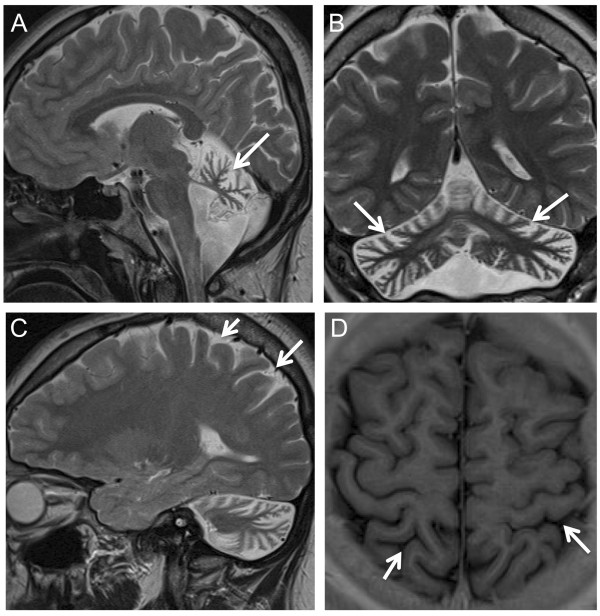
Cerebral Magnetic Resonance Imaging in *STUB1 *ataxia. T2 (A: sagittal; B, coronal; C, sagittal) and T1 weighted images (D, axial) of subject 18161 at age 17 years show marked atrophy of the cerebellar vermis (arrow, A) as well as the cerebellar hemispheres (arrows, B), and mild atrophy of the parietal cortex (arrows C, D).
